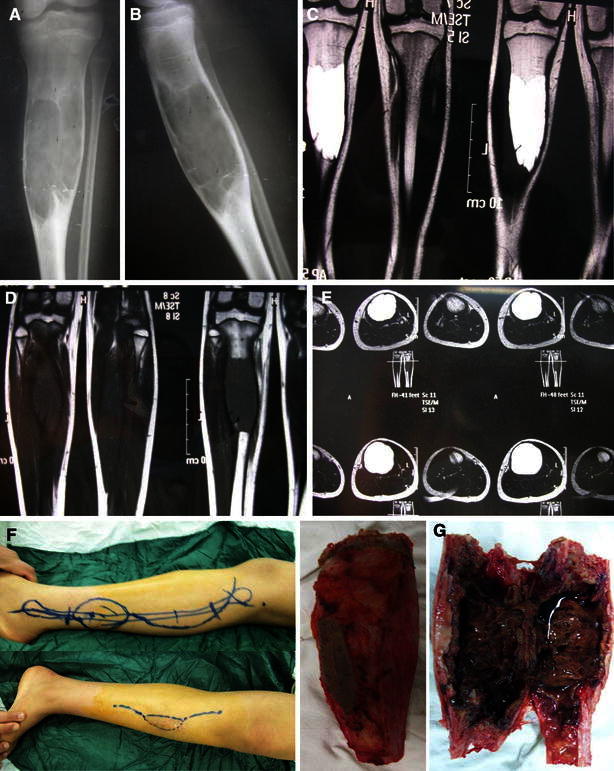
Operative technique for tumor resection and planning for fibula harvesting (18-year-old female patient with bone sarcoma of the tibia). a, b Preoperative plain X-ray; c–e preoperative MRI for diagnosis and staging; f, g tumor mass after resection
